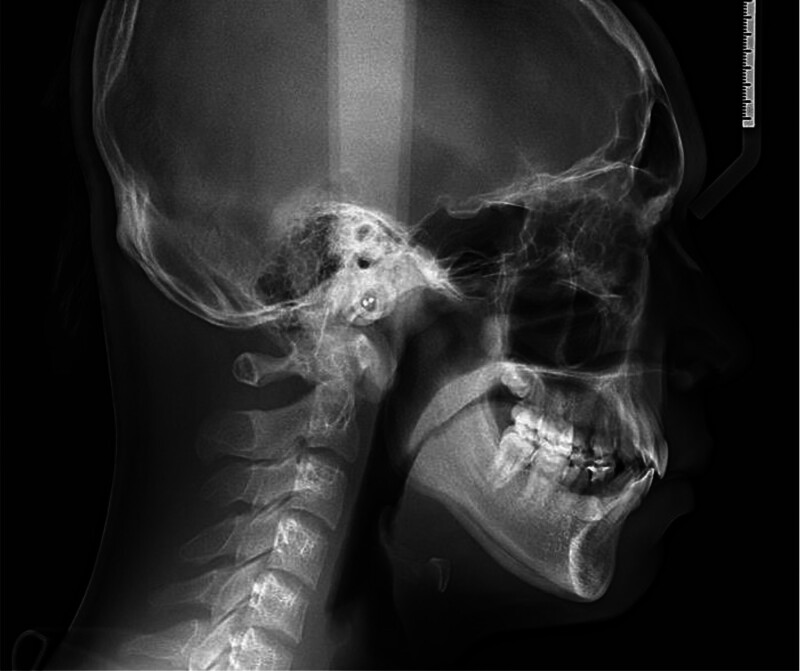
Pretreatment cephalometric radiograph.
